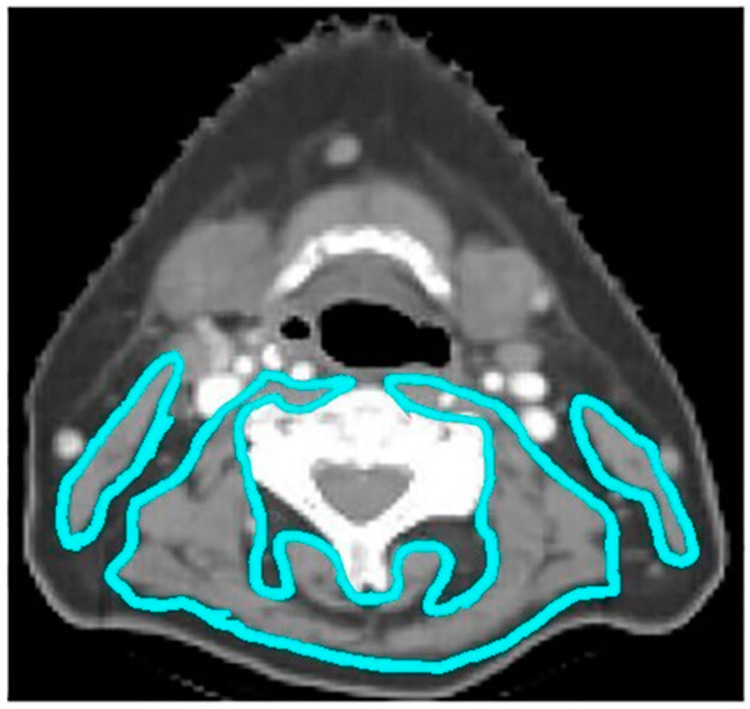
A representative case example of a 54-year-old man with an HPV-positive oropharynx cancer. A single axial CT slice is taken at the C3 vertebral body level with contours of the sternocleidomastoid and paravertebral muscles delineated in turquoise. Skeletal muscle was defined as −29 to +150 Hounsfield Units (HUs), and the total cross-sectional area (CSA) was computed automatically within the contoured perimeters.
